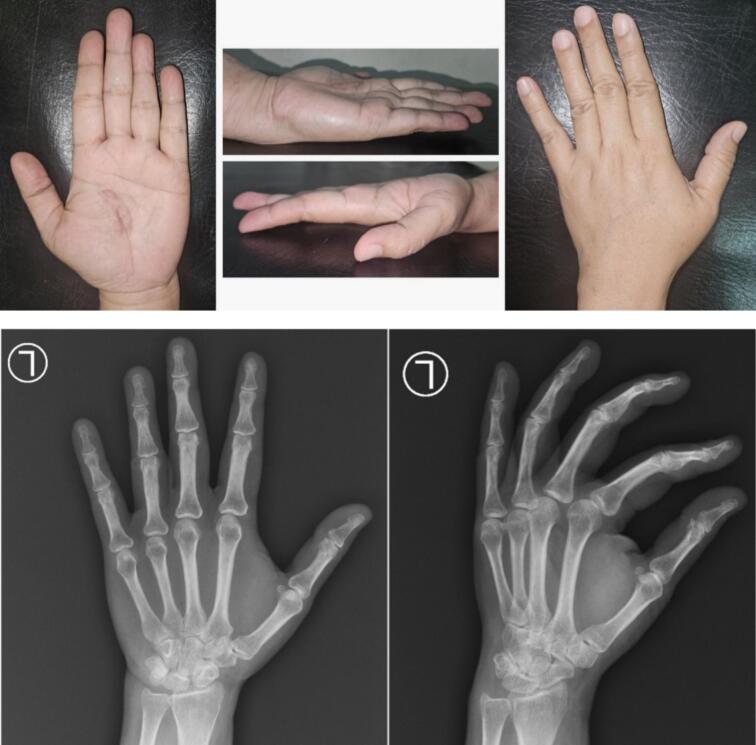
Clinical image and x-ray one month following surgical excision of osteolipoma.
